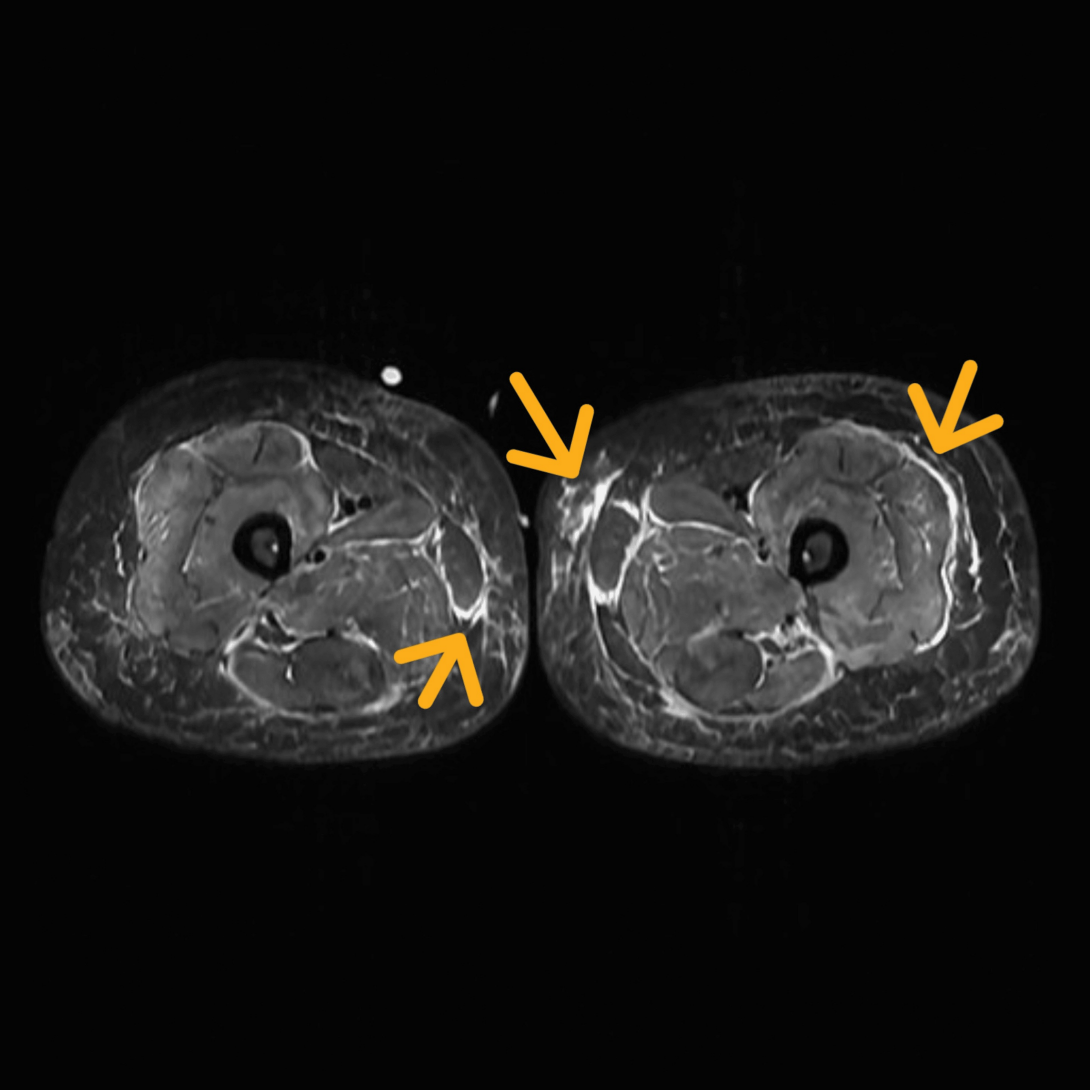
MRI of the muscles of both thighs STIR sequence showing hyperintensity in the thigh and pelvic girdle muscles (yellow arrows). STIR, short tau inversion recovery
